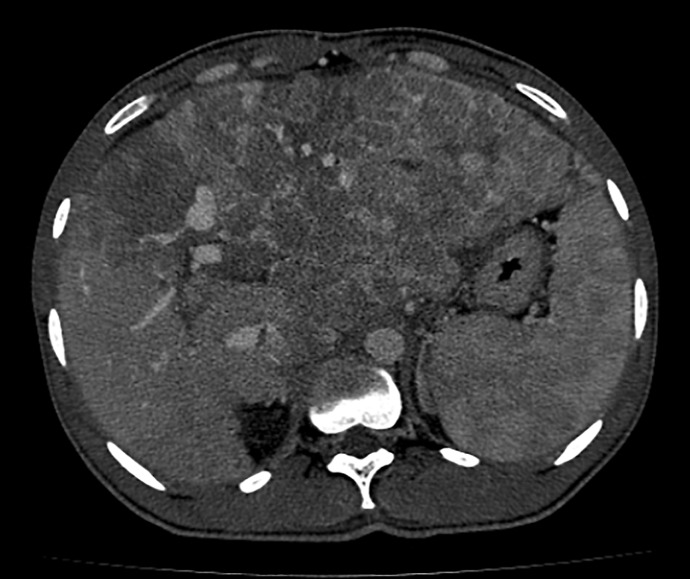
CT scan of the abdomen. Heterogeneous hepatomegaly with multiple coalescent lesions involving most of the left and caudate lobes and also the right lobe, with arterial enhancement and washout, suggestive of multicentric HCC. CT, computed tomography.
